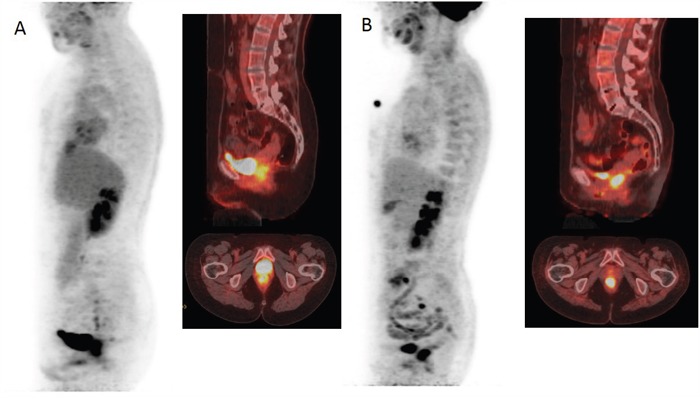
^18^F-FDG PET/CT images before A and after B treatment demonstrating a minimal reduction of glucose metabolism with ΔSUVmax of 6.07% classifying the patient as non responder.
